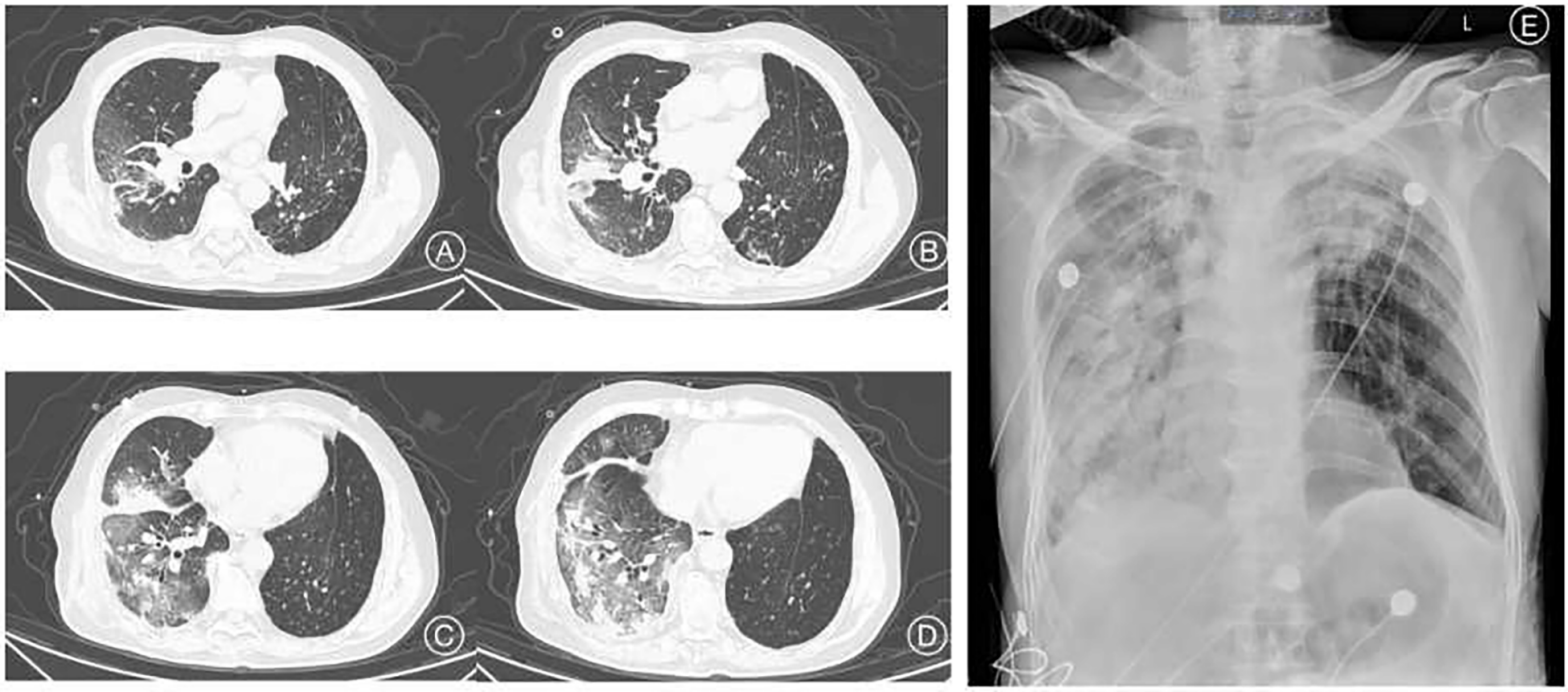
Clinical imaging of a 69-year-old man with pneumoconiosis, diagnosed before LTx and who underwent right single lung transplant surgery one and a half years before the COVID-19 episode. Chest CT images (A–D) show infiltration mainly involving the right transplanted lung. Chest radiograph image (E) 5 days after the CT evaluation. CT, computed tomography.
